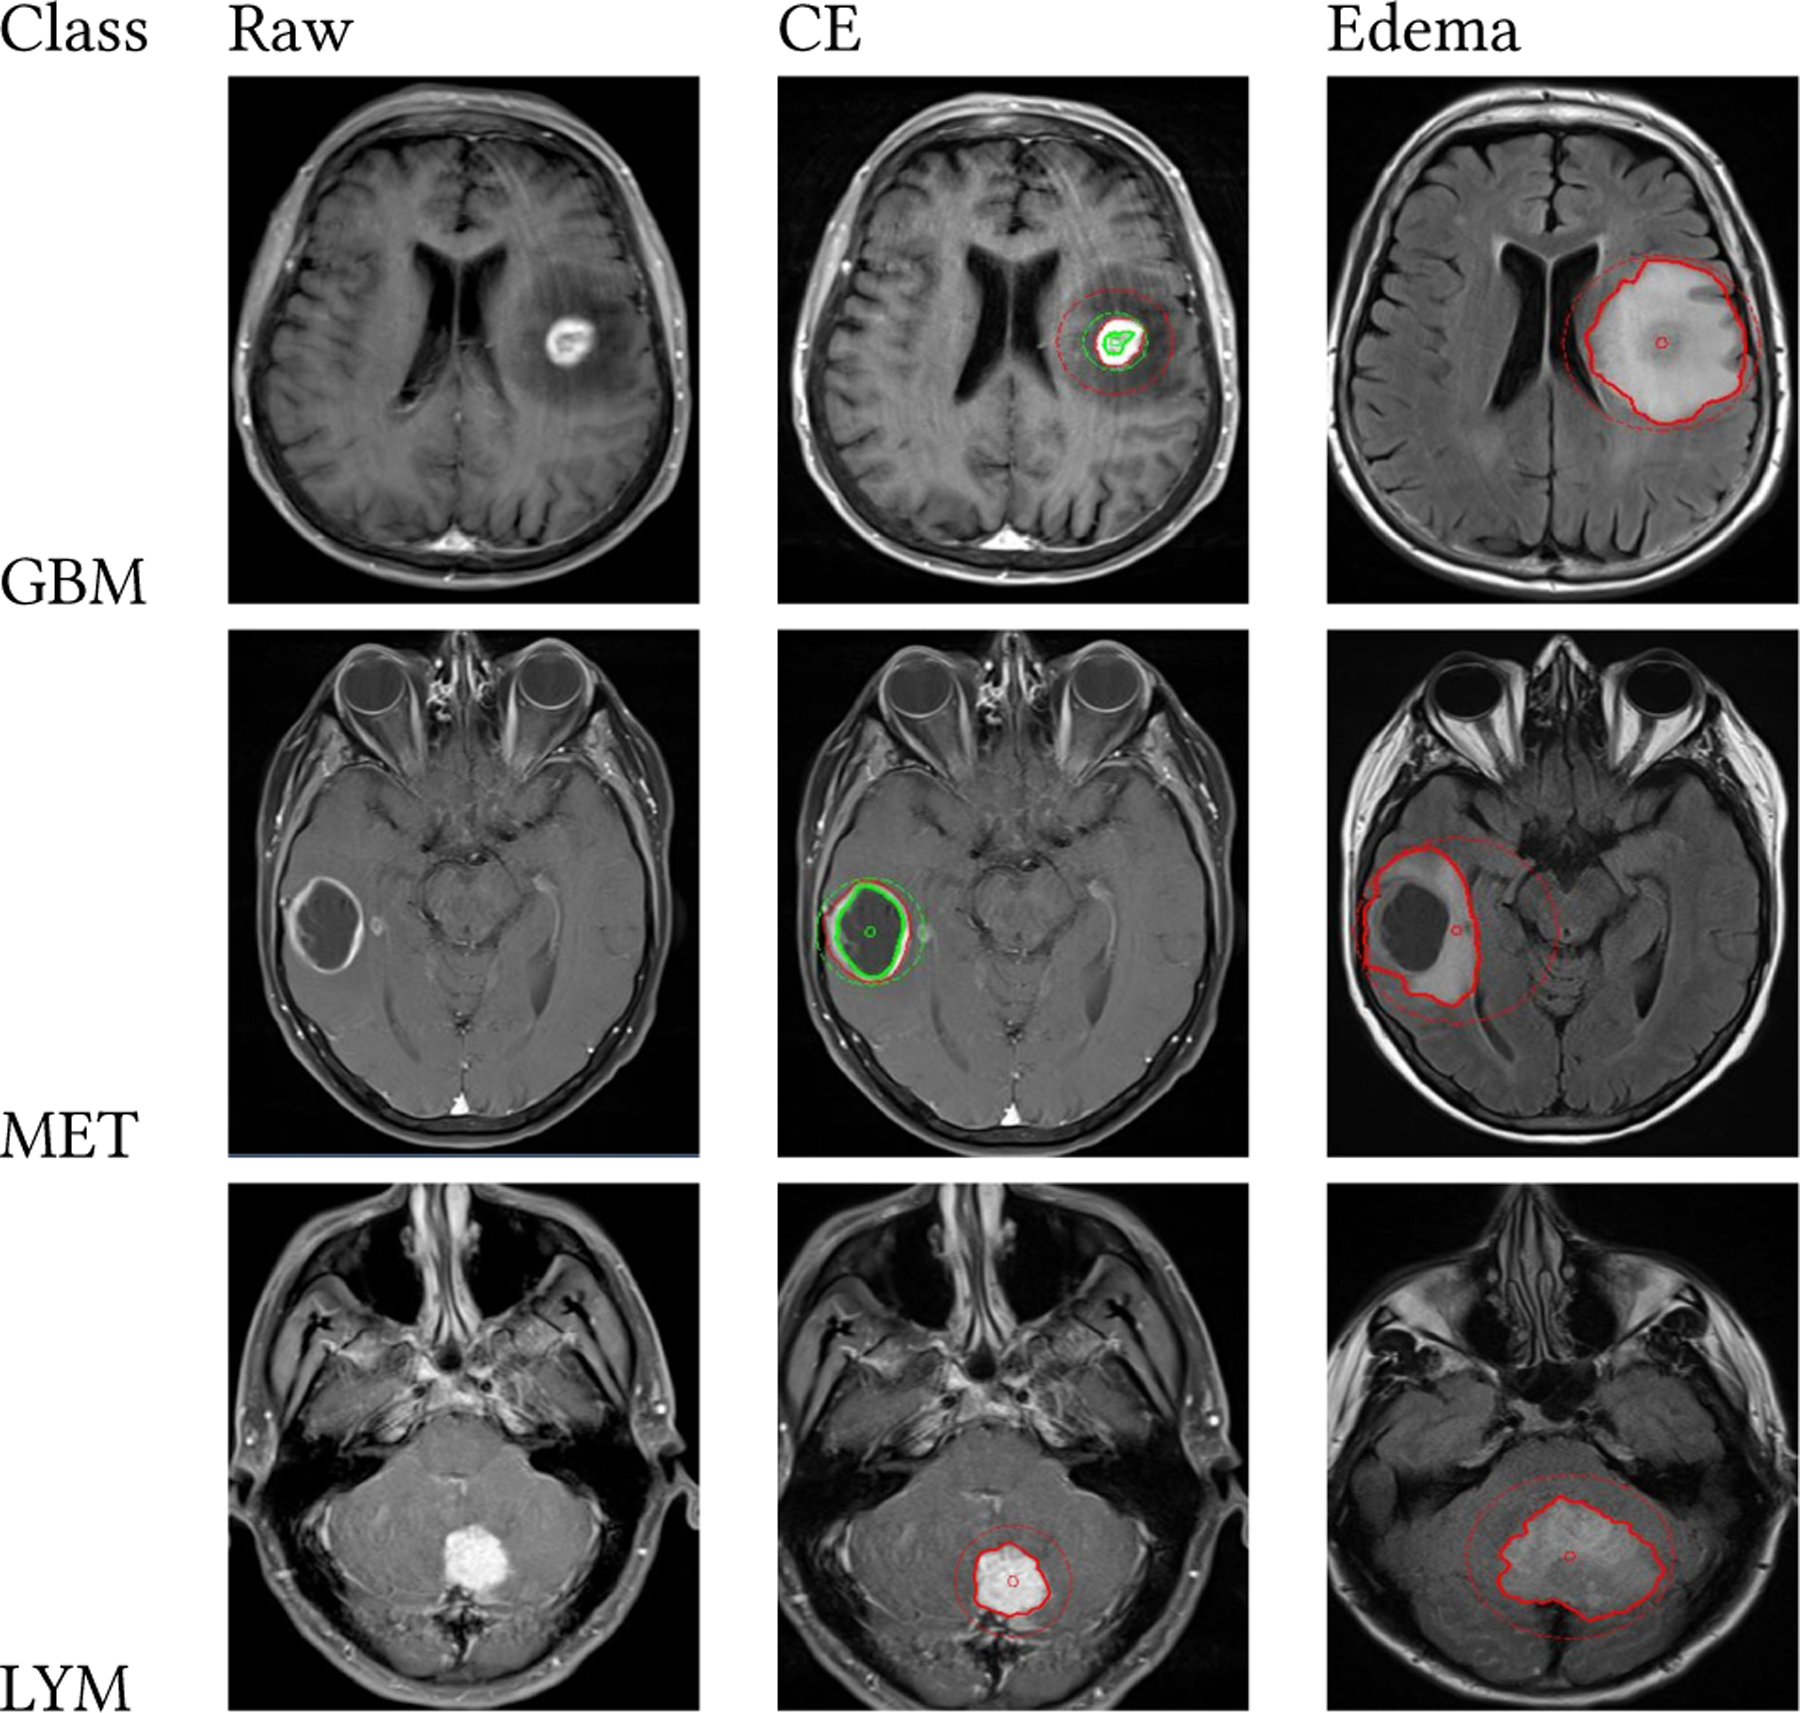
Example raw and contrast-enhanced MRI images and edema segmentation regions for each respective tumor class GBM, LYM, and MET.
